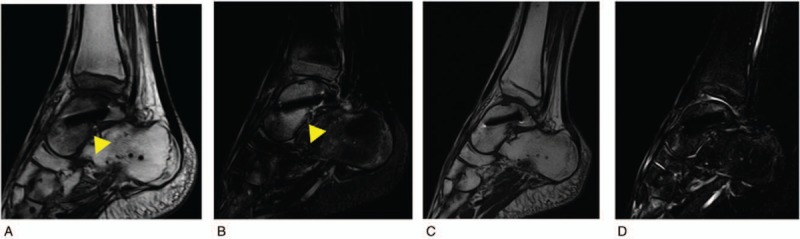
Sagittal images of magnetic resonance imaging (MRI) at 3 and 6 months after the surgery. (A) T1-weighted image at 3 months after the surgery. (B) T2-weighted short-tau invasion recovery (STIR) image at 3 months after the surgery. Dome of talus shows low intensity on T1-weighted image and high intensity on T2-weighted STIR image (arrow head). (C) T1-weighted image at 6 months after the surgery. (D) T2-weighted STIR image at 6 months after the surgery. Low intensity of the dome has changed to iso-intensity on T1-weighted image and high intensity on T2-weighted STIR image has also changed to iso-intensity.
